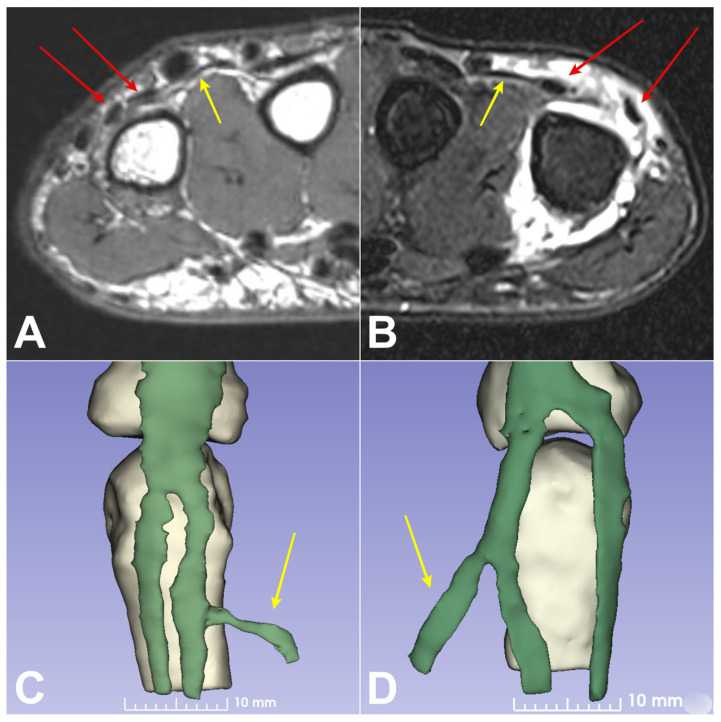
Representative MR images (case 1, 25-year-old male). Axial T1-weighted on the healthy side (A) and axial fat-suppressed T2-weighted with arthrography on the pathological side (B). MRI revealed a gap between the two tendon slips of the EDM (red arrows) on the pathological side. Using 3D reconstruction based on MRI, the maximum distance between the two tendons of the EDM was 7.2 mm on the injured side (D) and 1.5 mm on the contralateral side (C). The JT of the fourth inter metacarpal space (yellow arrow) was in continuity with the EDM on the pathological and contralateral side.
